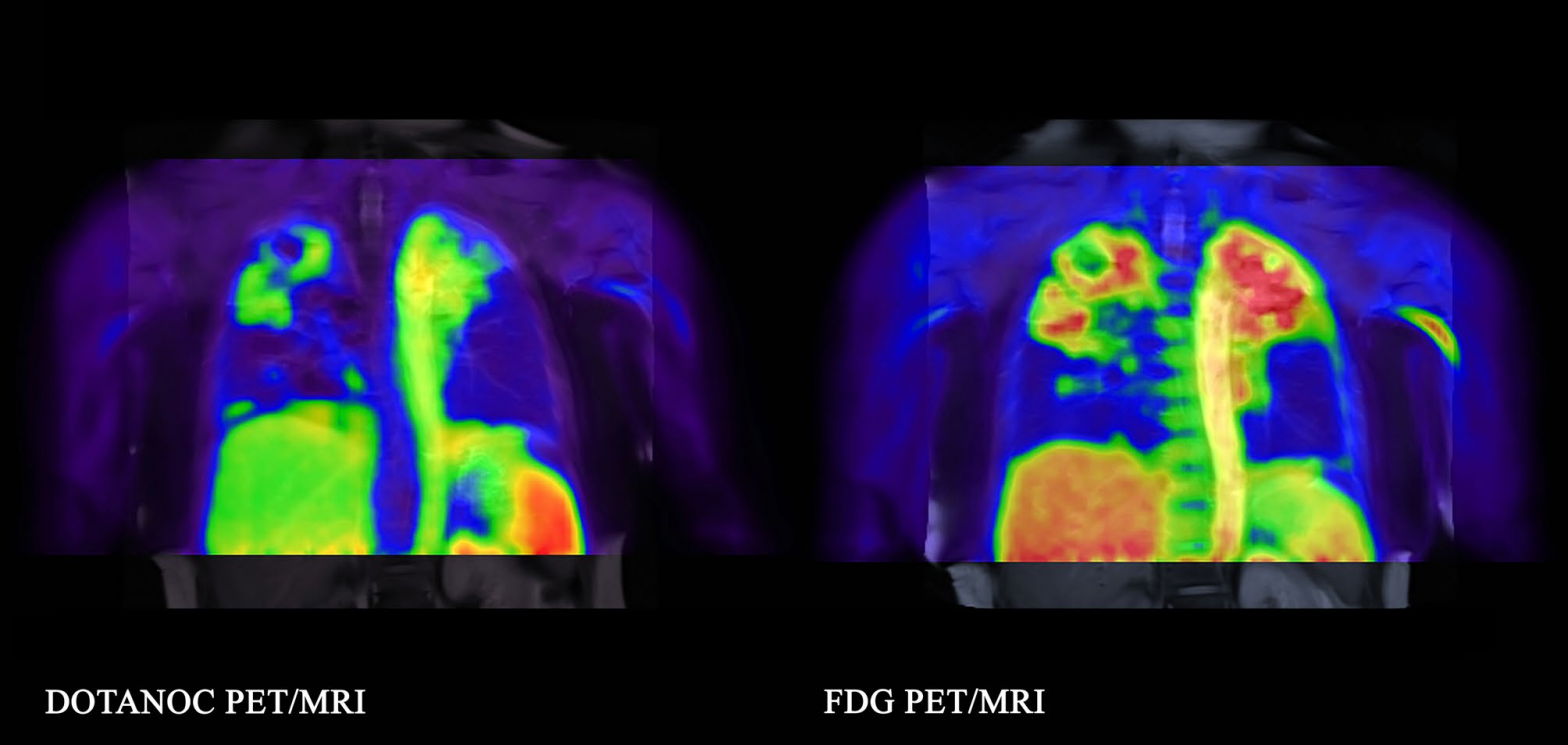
Example of diffuse uptake of FDG (SUVmax 8.22) compared to DOTANOC uptake (SUVmax 2.96) which is more discrete. 55-year-old woman with pulmonary TB, smear 3+, on Day 8 of TB treatment.
